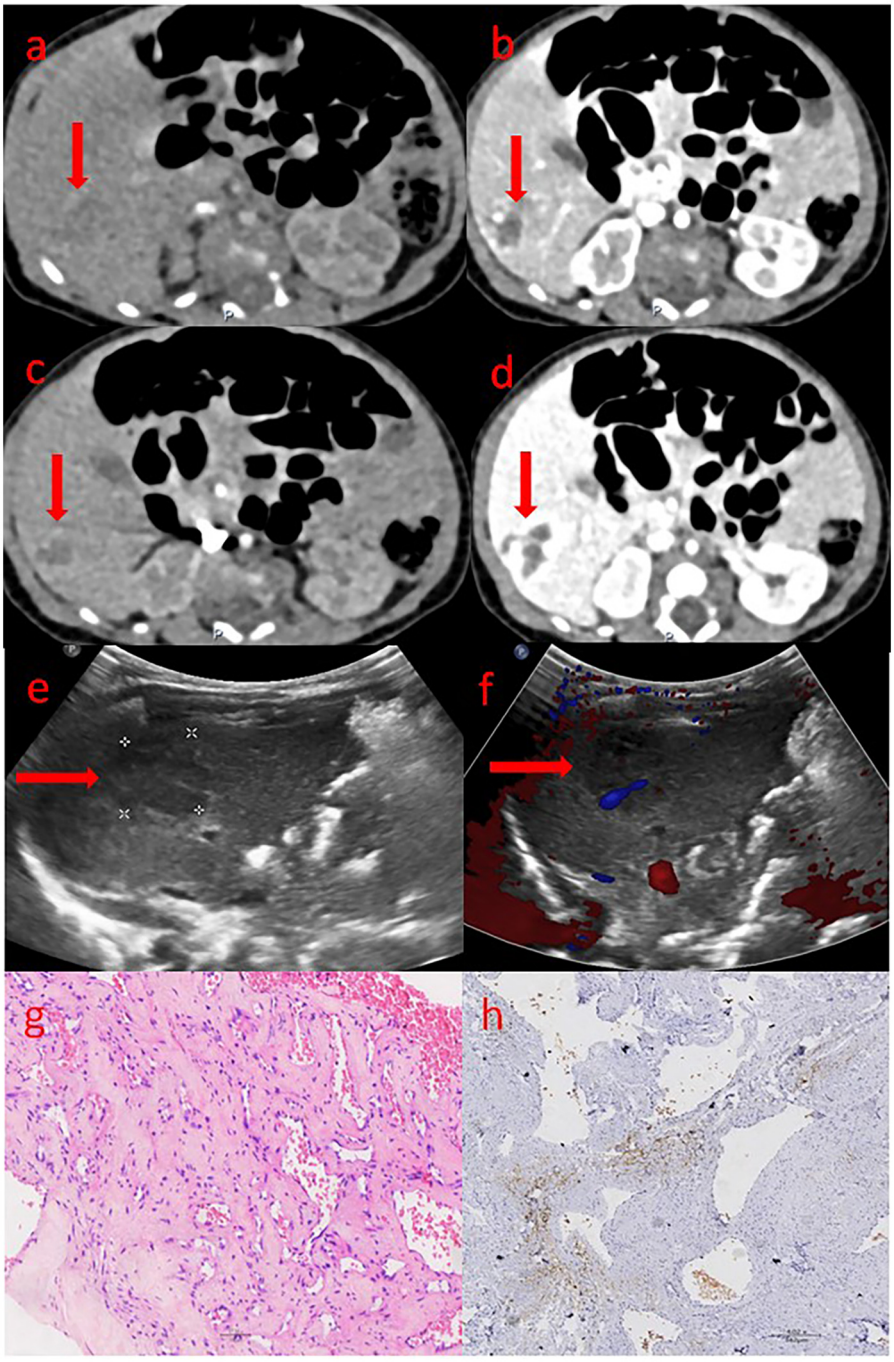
The ultrasound, CT and pathological images of congenital hepatic hemangioma. (a–d) shows the abdominal enhanced CT images: (a) Arterial phase; (b) Venous phase; (c) Portal phase; (d) Delay phase. The area marked by the arrow is the hemangioma. (e,f) shows the ultrasound images: (e) The two-dimensional gray-scale image; (f) The color Doppler image. The area marked by the arrow is the hemangioma. (g,h) shows the pathological images: (g) The tumor tissue shows varying degrees of vascular proliferation, dilation and congestion, as well as proliferation of fibrous tissue around the vessels. (h) endothelial cells do not express GLUT-1, red blood cells can be used as a positive internal control.
